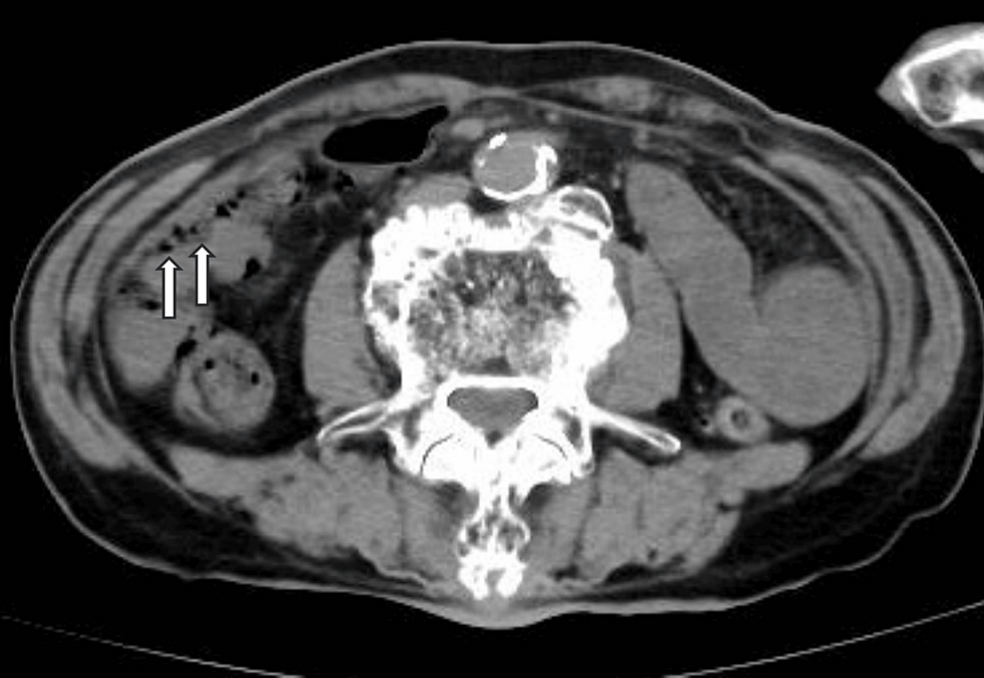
Intramural emphysema of the ileum shown on abdominal CT
